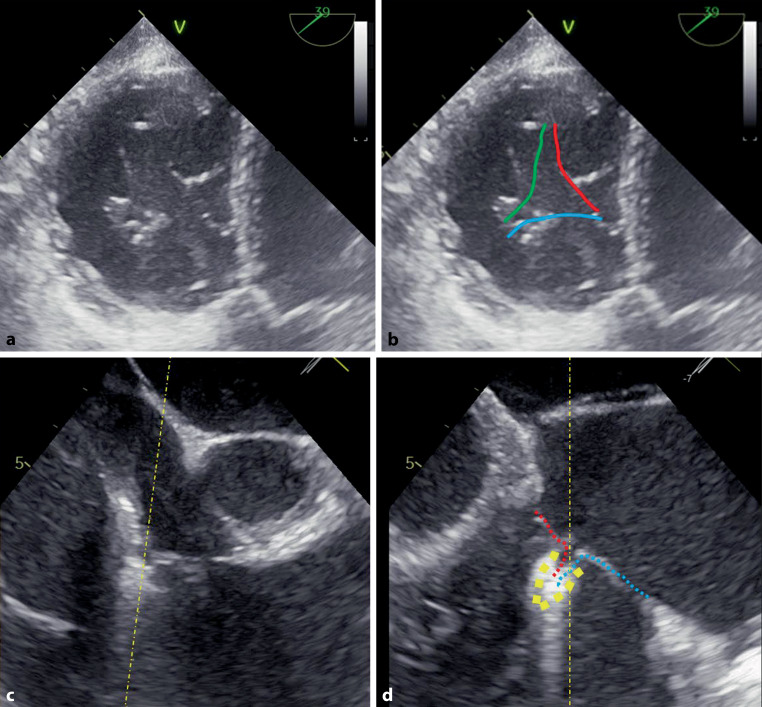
a–c Visualisation of tricuspid valve clipping by transoesophageal echocardiography. a Transgastric short axis view of the tricuspid valve. b Same view as in (a); the three leaflets are highlighted: *red* septal, *green* posterior, *blue* anterior. c and d Cross-plane image in mid-/deep oesophageal position showing the septal (*red*) and anterior (*blue*) leaflet positioned in a closed clip (*yellow*)
